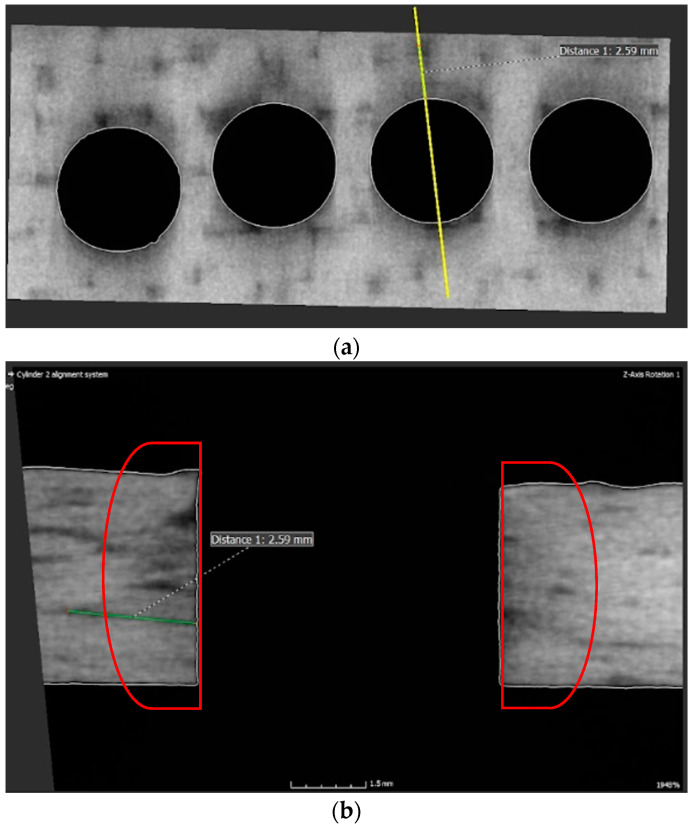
CT images of drilled holes from computed tomography. The evaluated sample (yellow highlighted cut point) (a) with a visible area of delamination. Display of delamination inside a hole, indicating the extent of material damage due to delamination (b).
